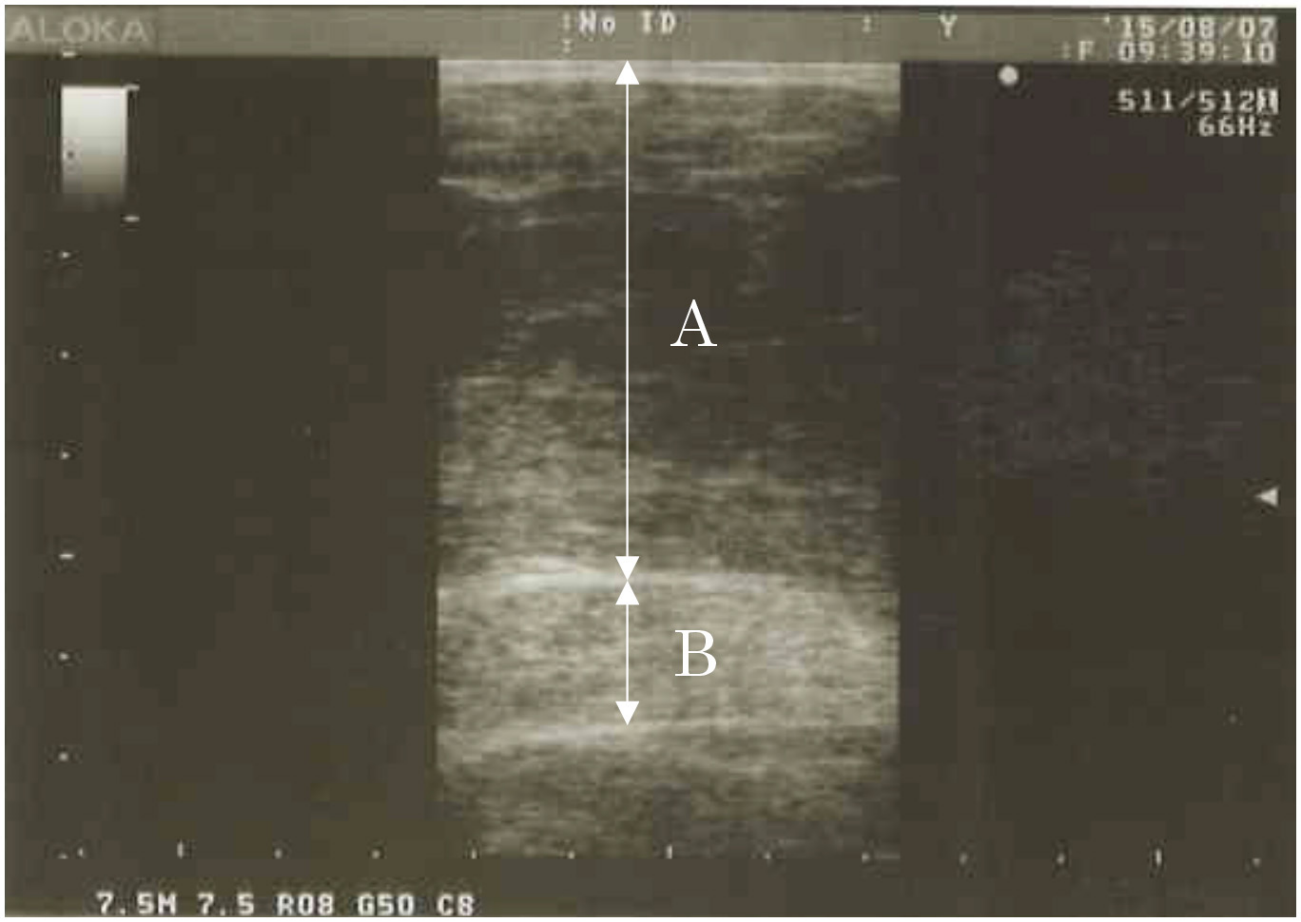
Typical abdominal ultrasound image in obesity patient. The thickness values of the muscle tissues was determined as the distances between the skin and fat—muscle tissue interface and between the fat—muscle tissue interface and muscle—abdominal cavity boundary. (A) Subcutaneous fat thickness, (B) Muscle thickness.
